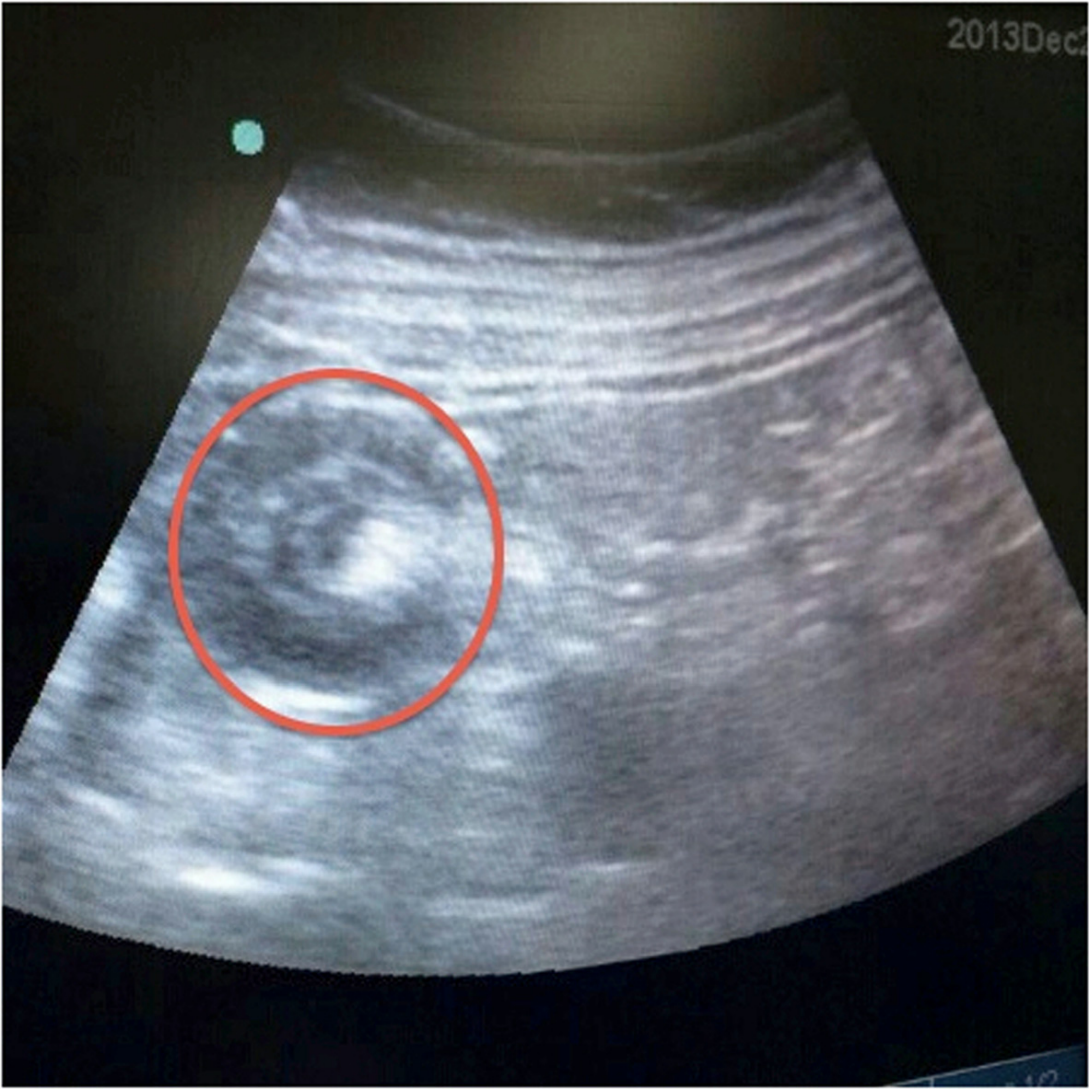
An intraoperative ultrasound, showing the classic target sign appearance of intussusception.
